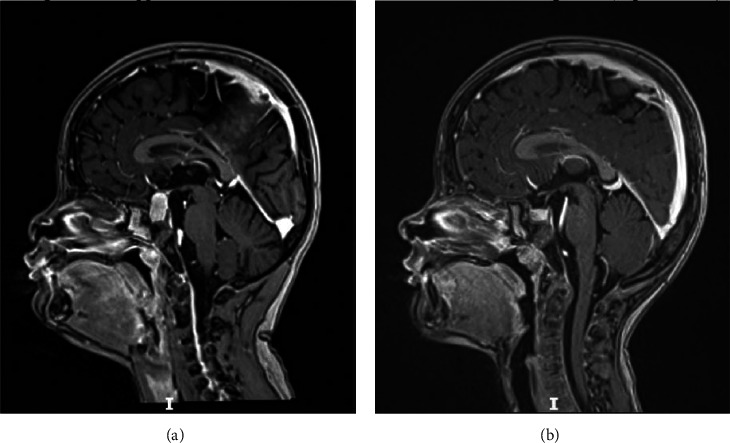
Magnetic resonance imaging (MRI) sagittal T1-weighted image sequences. (a) Pituitary hyperplasia (diameters 18 × 12 × 17 mm, convex upper margin). (b) Regression of pituitary dimensions 6 months after presentation.
